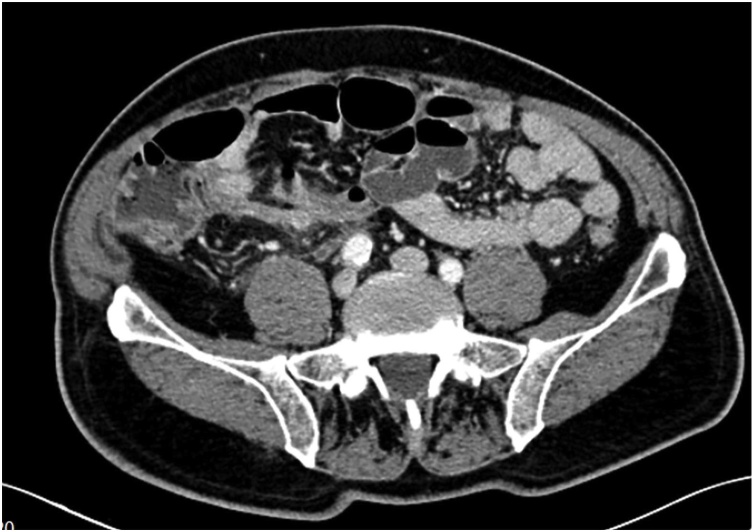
Abdominal CT scan showing acute appendicitis.
